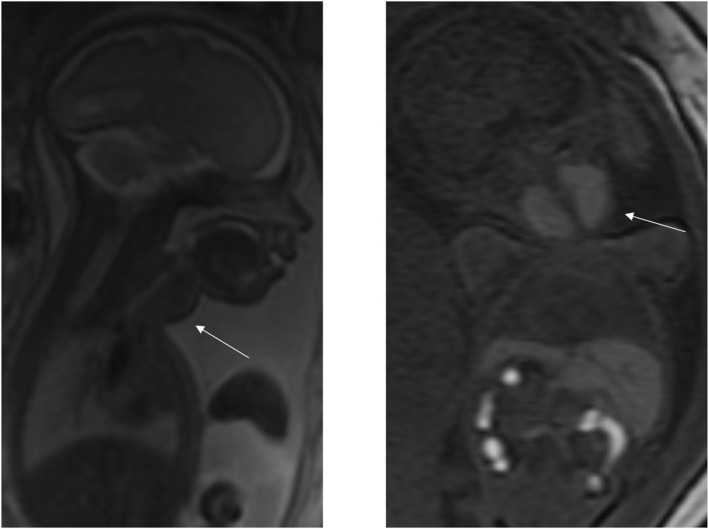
Fetal MRI images of a goiter. Left image ‐ T2 SSFSE sagittal image of 21 weeks fetus showing a solid homogeneous anterior neck mass. Right image ‐ T1 coronal image of the same fetus showing increased signal in the thyroid (goiter) and the meconium in the bowel.
